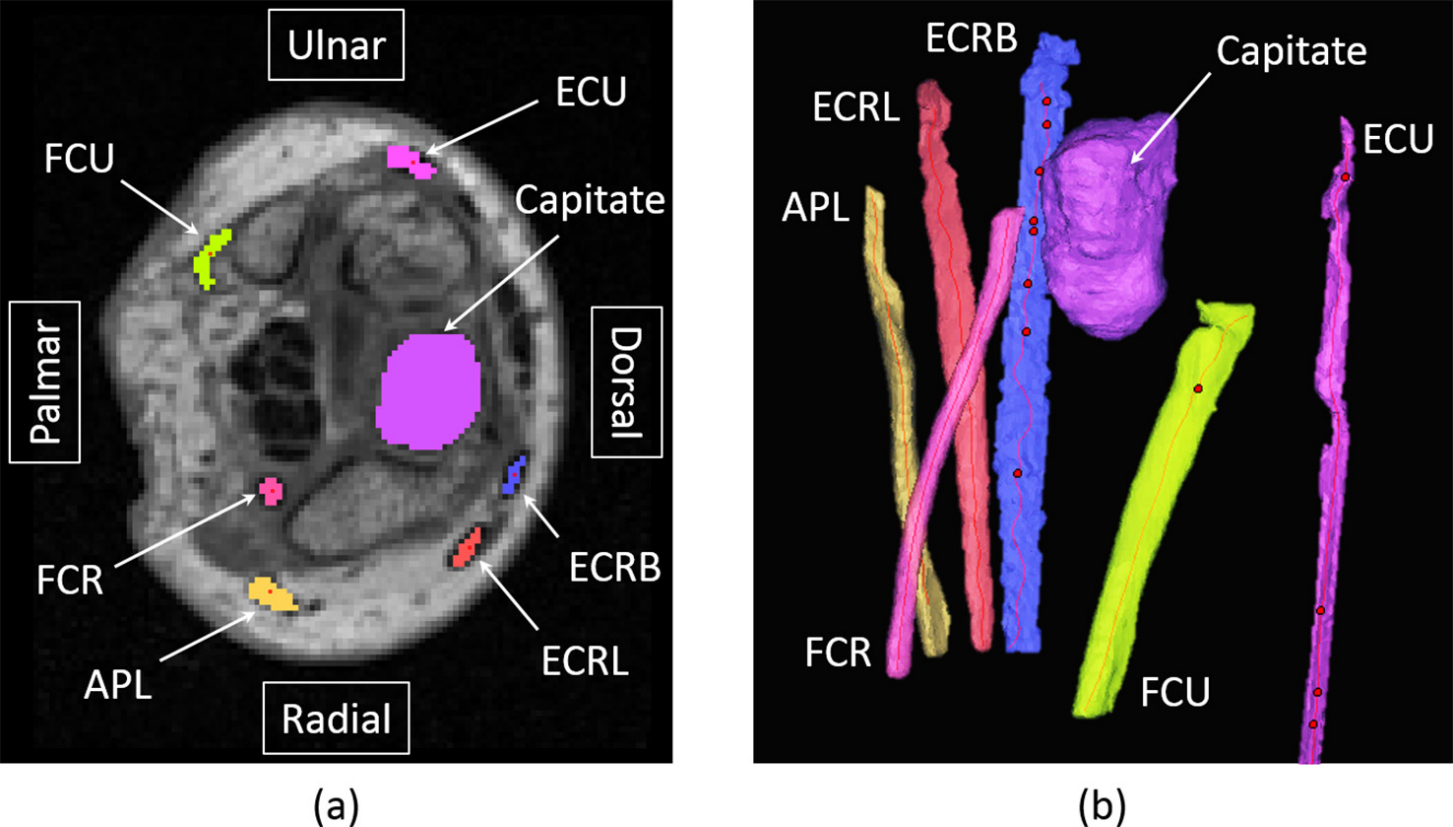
The capitate and tendons of the wrist, with centrelines, as seen in (a) a transverse section of a magnetic resonance imaging (MRI) scan and (b) 3D reconstructions from the MRI scan. Moment arms of the tendons of the flexor carpi radialis (FCR), flexor carpi ulnaris (FCU), extensor carpi radialis longus (ECRL), extensor carpi radialis brevis (ECRB), extensor carpi ulnaris (ECU), abductor pollicis longus (APL) were quantified.
